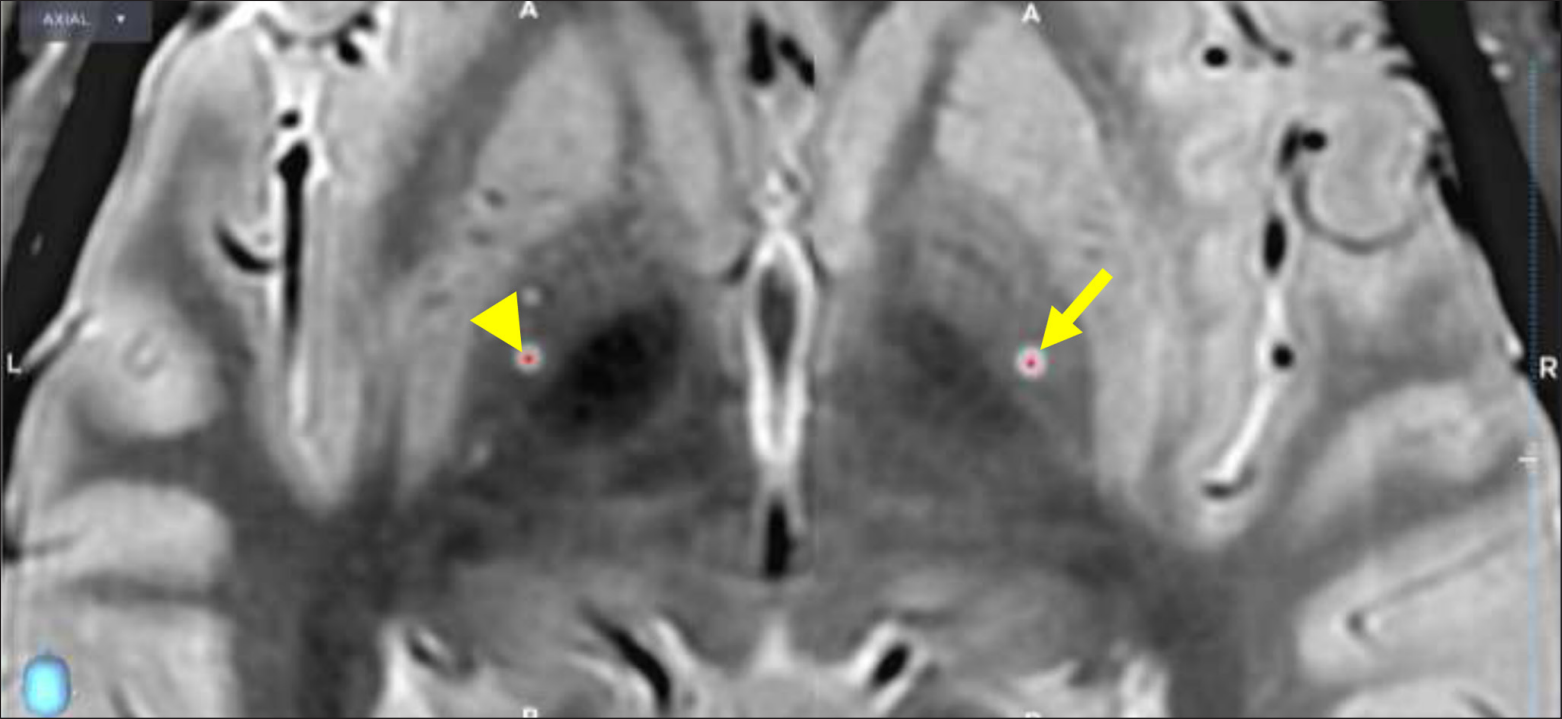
An intraoperative CT scan, taken immediately after bilateral lead placement, is merged with the preoperative MRI to assess stereotactic error and to visualize the contact location relative to the surrounding anatomy. Visible in this figure are contact 2 on the left (i.e., third contact from the bottom, arrowhead) and contact 9 on the right (i.e., second contact from the bottom, arrow). Contact 2 is centered at (–20.86, 4.04, –1.25), representing a radial error of 0.2 mm off of the surgical plan. Contact 9 is centered at coordinates (21, 4.25, –0.57), representing a radial error of 0.3 mm off of the surgical plan. Both contacts are positioned medial to the border between the globus pallidus interna and the globus pallidus externa.
